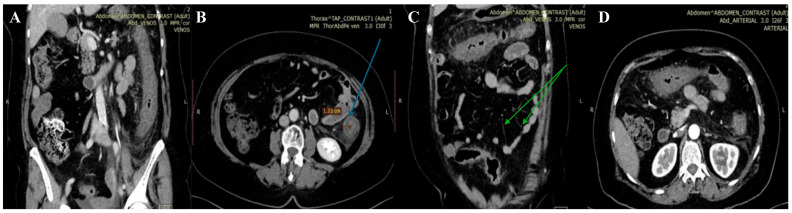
First abdominal CT. Spastic large intestine, with thickened circumferential mucosa (blue arrow), up to 1.33 cm (B), and an incomplete “comb” sign (green arrow) (C). Coronal (A,C) and axial planes (B,D) respectively.
